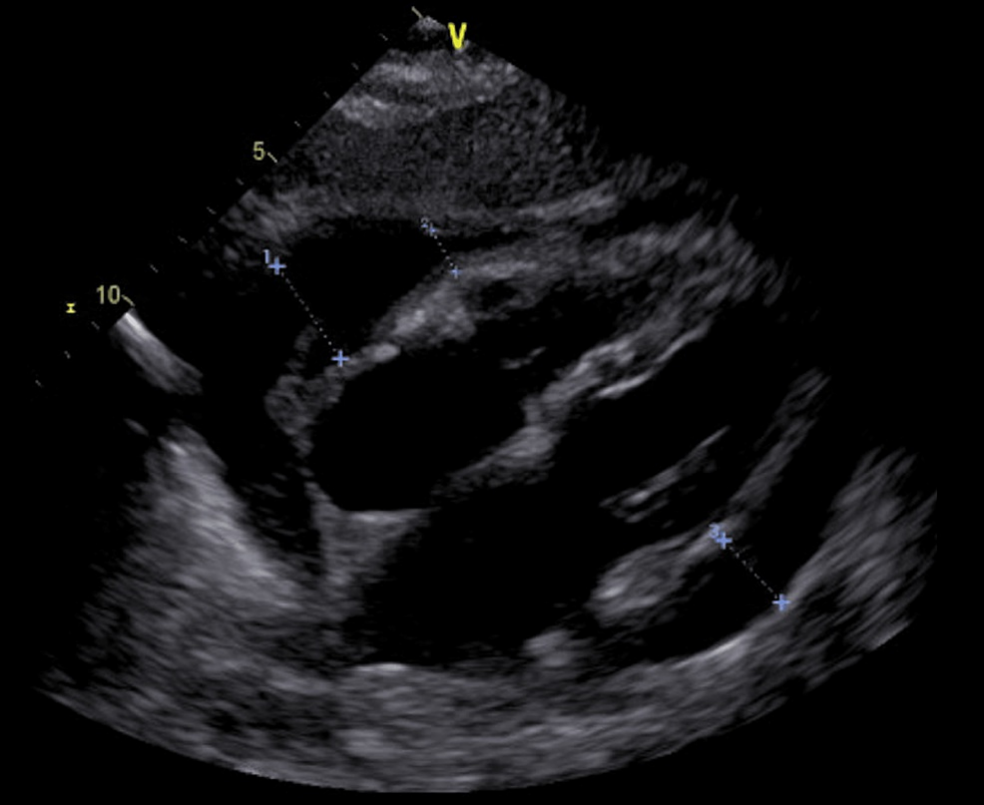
Echocardiogram showing moderate to severe collection of pericardial fluid
